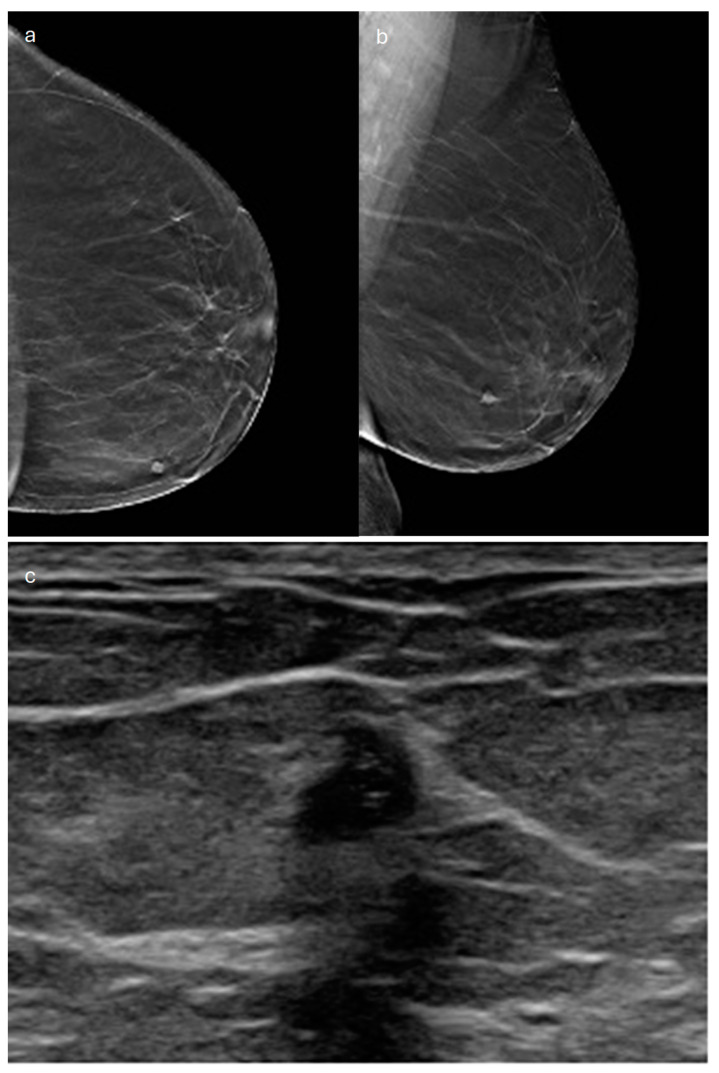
Apocrine carcinoma in a 61-year-old patient. (a) Cranio-caudal and (b) medio-lateral oblique DBT images of the left breast show a small, irregularly shaped opacity in the lower-inner quadrant (c) corresponding to a hypoechoic mass with non-circumscribed margins on ultrasound.
